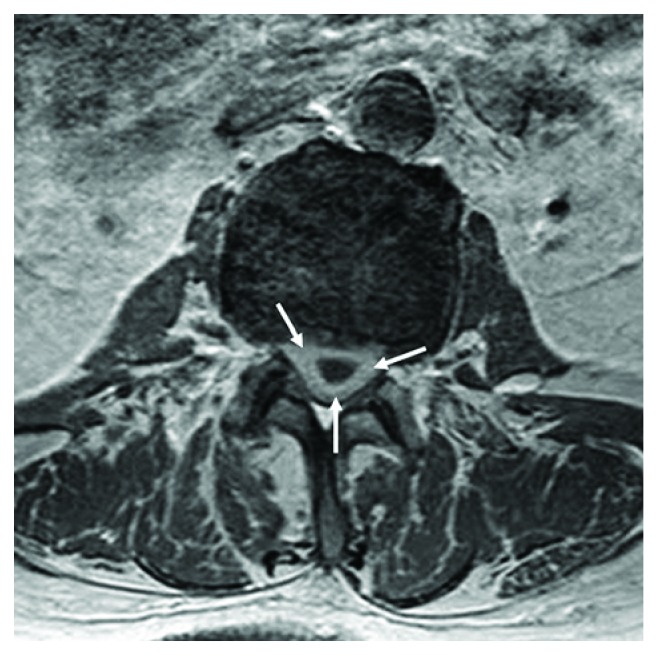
Axial T1-weighted MRI with contrast demonstrated hyperintense lesion periphery.
